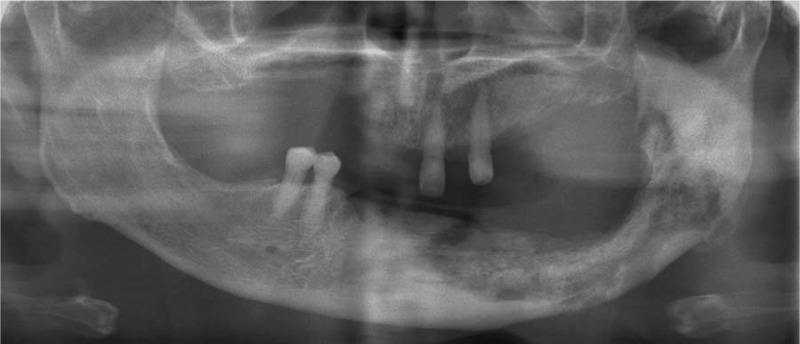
Orthopantomography 3 weeks before hemimandibulectomy.
